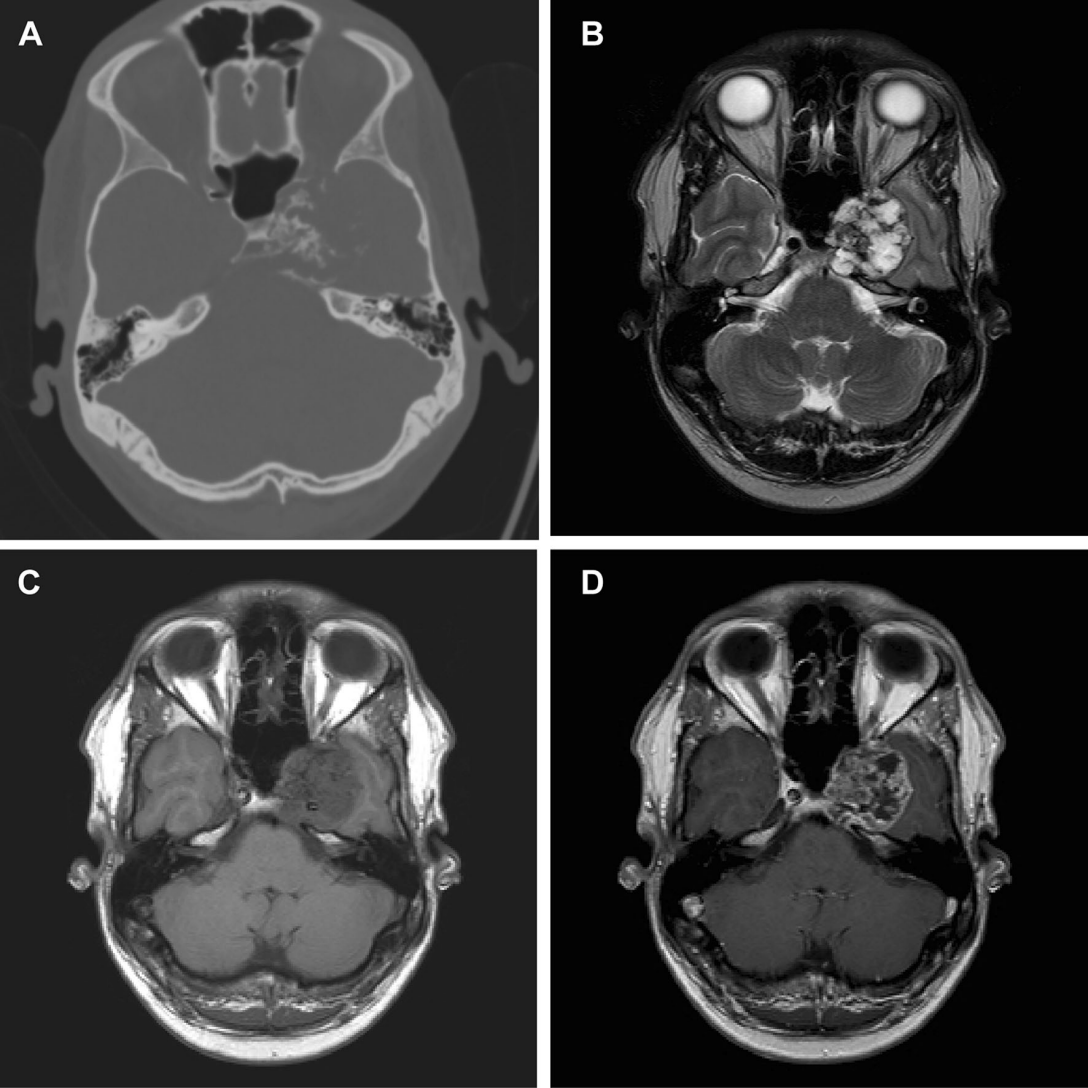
Chondrosarcoma. Imaging of skull base chondrosarcoma arising from the left lateral clivus with extension to the clinoid process, cavernous sinus and intracranial extension and compression on the left temporal lobe. Computed tomography (a) shows bone destruction with hyperdense fragments in the tumour mass, which corresponds with the typical ‘rings-and-arcs’ seen in chondroid tumours like chondrosarcoma. T2-weighted imaging (b) shows a heterogenous, mostly hyperintense mass. T1-weighted imaging (c) shows a hypointense mass with heterogenous enhancement (d). Pathologic examination of the tumour mass concluded chondrosarcoma WHO grade 1
